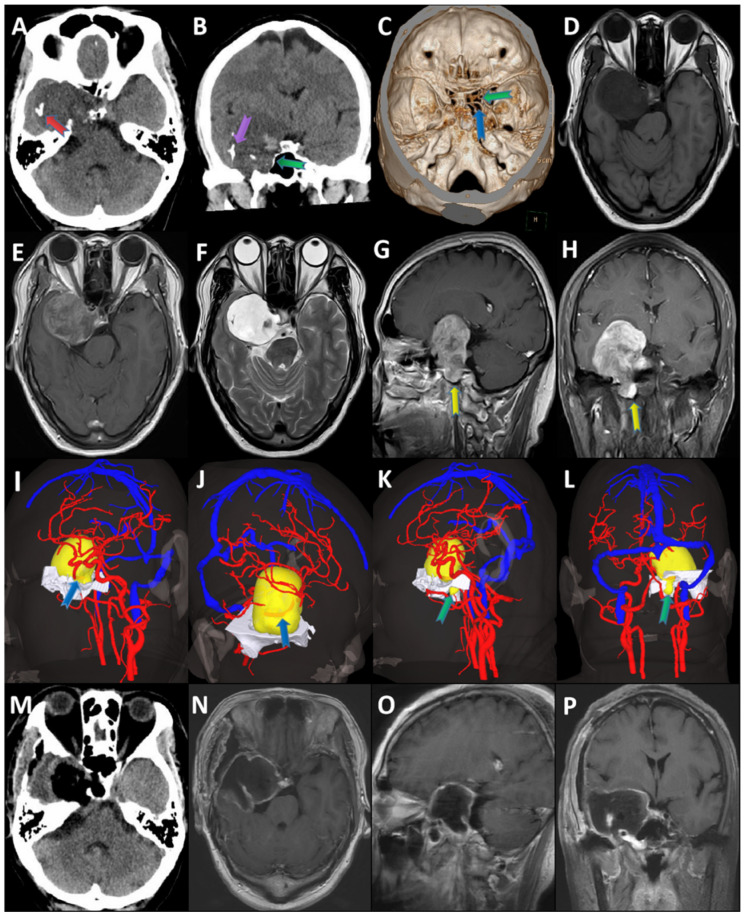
The preoperative, intraoperative, and postoperative images of right parasellar chondrosarcoma were displayed. (A): Preoperative CT scan showed mixed density shadow near the right parasellar, the position indicated by the red arrow in (A) is the bone composition in the tumor; (B,C): preoperative CT bone window (B) and CTA imaging reconstruction (C) showed that the bone destruction (indicated by the green arrow) and osteogenesis (indicated by the purple arrow) can be seen before operation, while important arteries were compressed by the tumor (indicated by the blue arrow); (D–H): the main body of tumor showed long T1 on T1-weighted image MRI before operation in (D), the position indicated by the yellow arrow in (G,H) showed tumor surrounded extracranial, preoperative T2-weighted image MRI showed that the tumor was long T2 in (E,G,H,F): MRI enhancement showed that the tumor was significantly enhanced; (I–L): MRI 3D reconstruction, the position indicated by the blue arrow in (I,J) showed internal carotid artery surrounded by tumor, the position indicated by the green arrow in K and L showed tumor surrounded extracranial; (M): after operation, CT plain scan showed low density shadow near the right parasellar, which was normal after operation; (N–P): right parasellar tumor was excised on T1 and T2 MRI after operation.
